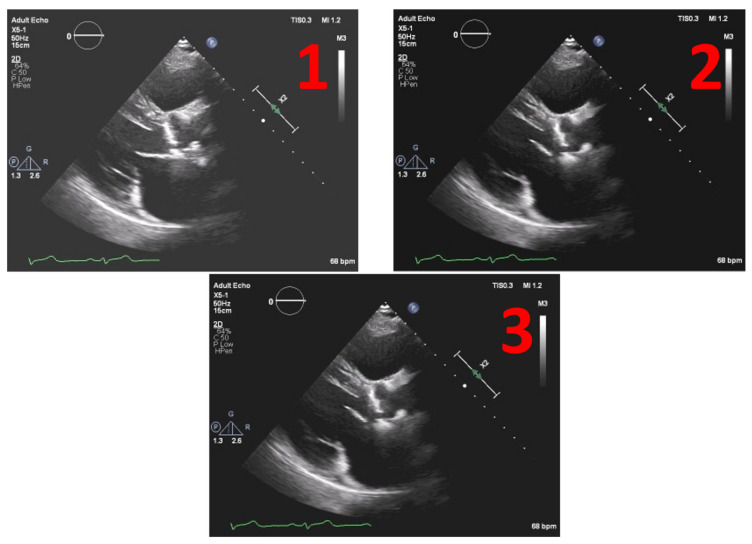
Echocardiography examples with different Windrow Levels (WL) and fixed Window Width of 250 (1) WL = 75, (2) WL = 100, and finally, (3) WL = 125.
